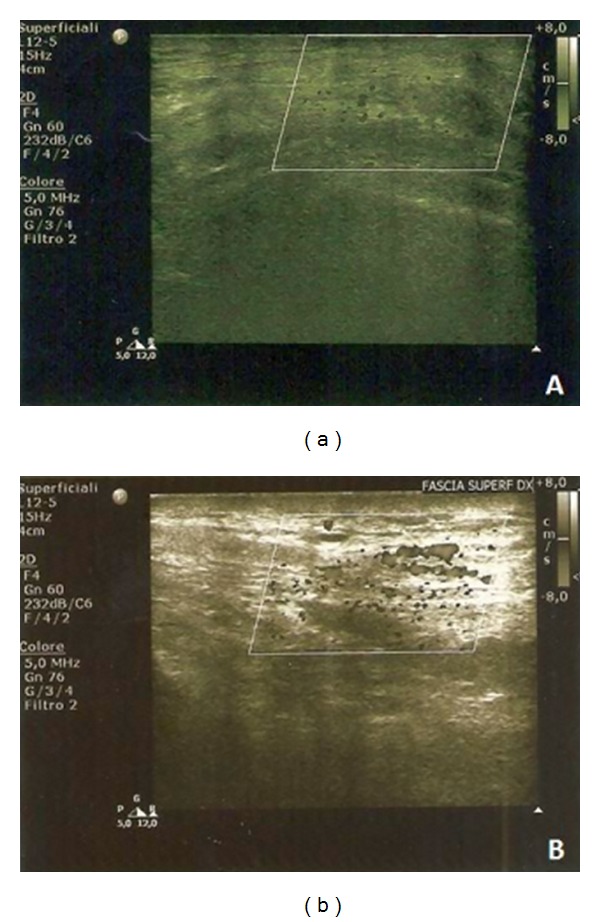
(a) Preoperative Doppler ultrasonography (V0); (b) 3-month postoperative Doppler ultrasonography (V1).
